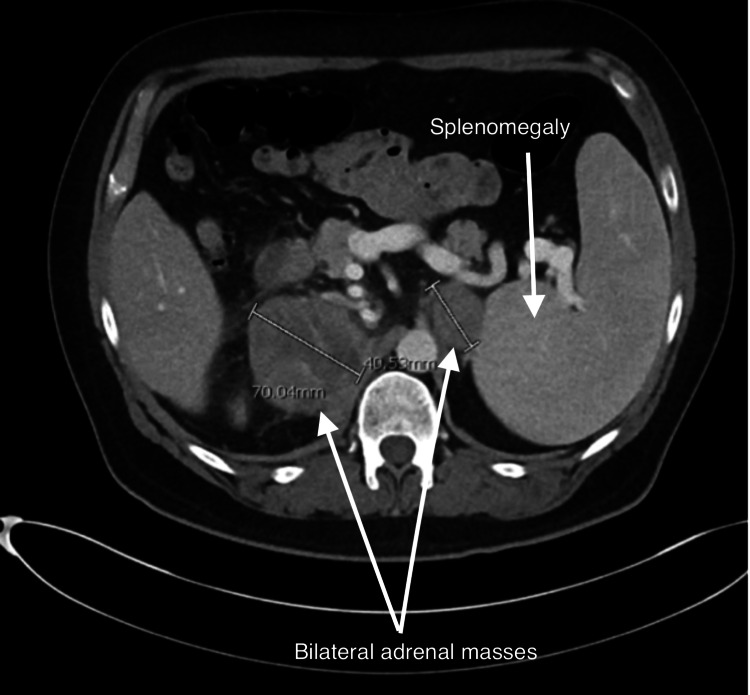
CT abdomen and pelvis with contrast Bilateral adrenal masses (6.7 cm right, 4.5 cm left), indistinct from adrenal glands. Splenomegaly (18 cm), no focal splenic lesion, with high Hounsfield units >20.
